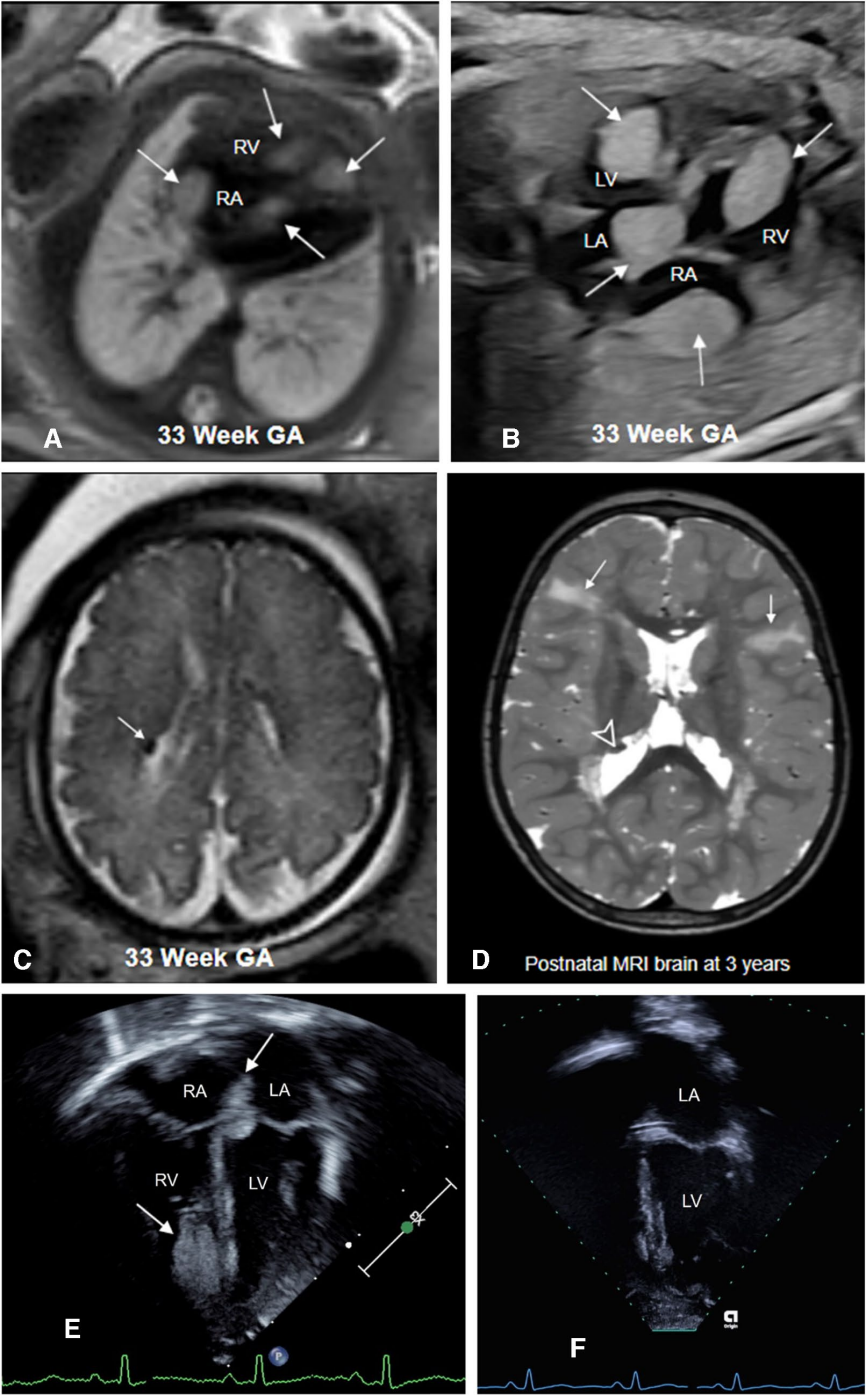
A 33-week gestational age (GA) fetus with confirmed TSC2 gene mutation. A Axial T2-weighted image shows multiple T2 hyperintense ovoid lesions (*arrows*) involving the myocardium and inter-ventricular septum. B Four-chamber echocardiography image demonstrating multiple ovoid hyperechoic masses (*arrows*) in the same location as the MR. C Axial balanced steady state free precession (bSSFP) brain image showing a hypointense subependymal nodule (*arrow*) along the right lateral ventricle. The patient received mTOR inhibitors. D Axial T2-weighted image of the brain at 3 years of age showing multiple cortical, subcortical lesions/tubers (*arrows*), and subependymal nodules (*arrowhead*) typical of tuberous sclerosis. Postnatal echocardiogram in an apical 4-chamber view confirmed the presence of rhabdomyomas (*arrow*) in the same locations identified prenatally (E), but over time they reduced in size, with the most recent echocardiogram performed at 5 years of age showing no intra-cardiac tumors (F). *LV*, left ventricle; *RV*, right ventricle; *LA*, left atrium; RA, right atrium
